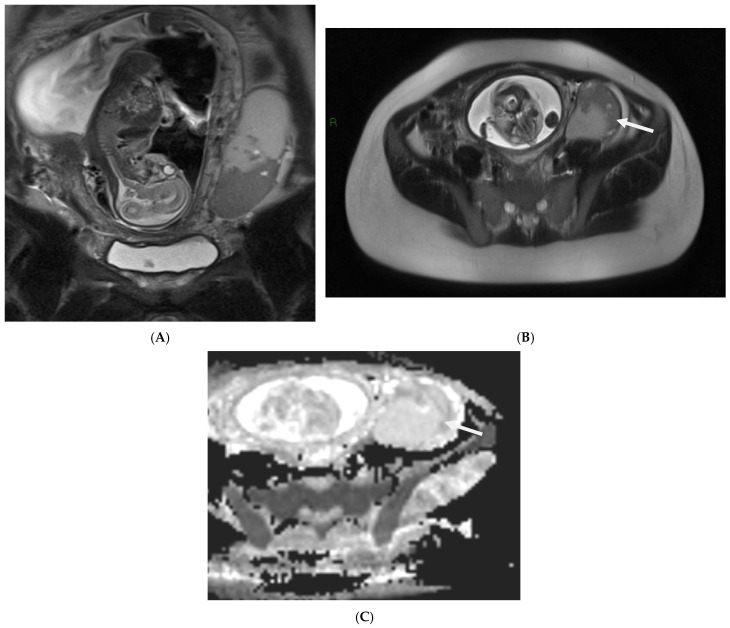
A 36-year-old woman at gestational week 32. Coronal (A) and axial (B) T2W Haste MR images show a large multilocular cystic mass in the left ovary. The signal intensity of the fluid varies, producing heterogeneous signal intensity, with suspected mural solid components (arrow). (C) Axial ADC map shows the mural nodule (arrow) with a relatively high ADC. A mucinous ovarian borderline tumor was found at surgery, performed after delivery.
